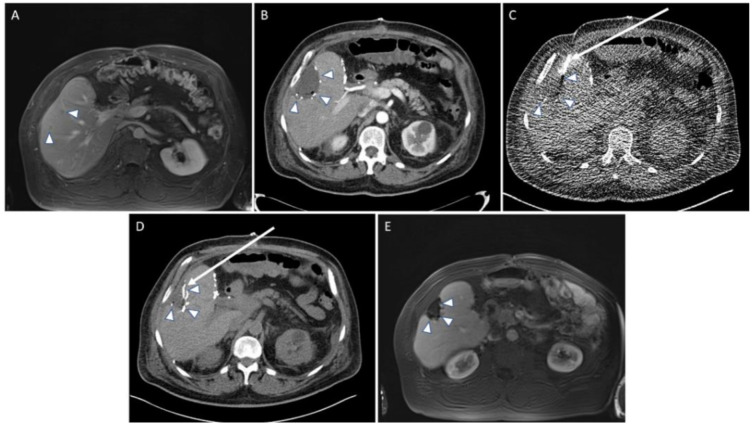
Example of a regular procedure of a CT-guided drainage placement. (A) A 64-year-old man with a history of neuroendocrine tumor of the ileum with liver metastasis and previous left side hemihepatectomy. MRI follow-up with hepatobiliary contrast media one year later revealed two new small metastasis in segments 5 and 8 (arrowheads). (B) The patient developed fever 5 days after the atypical resection of the liver segments 5 and 8. CT revealed fluid collection and small gas bubbles in the resection cavity, indicating an abscess formation. Arrowheads: resection margins. (C) CT fluoroscopic image with drainage (arrow) placement. Arrowheads: resection margins. (D) Post-interventional CT control scan. After placement of a 10 F drainage (arrow) and aspiration, the size of the fluid collection becomes significantly smaller. Arrowheads: resection margins. (E) MRI follow-up six months later showed unsuspicious resection margins (arrowheads) with granulation tissue.
